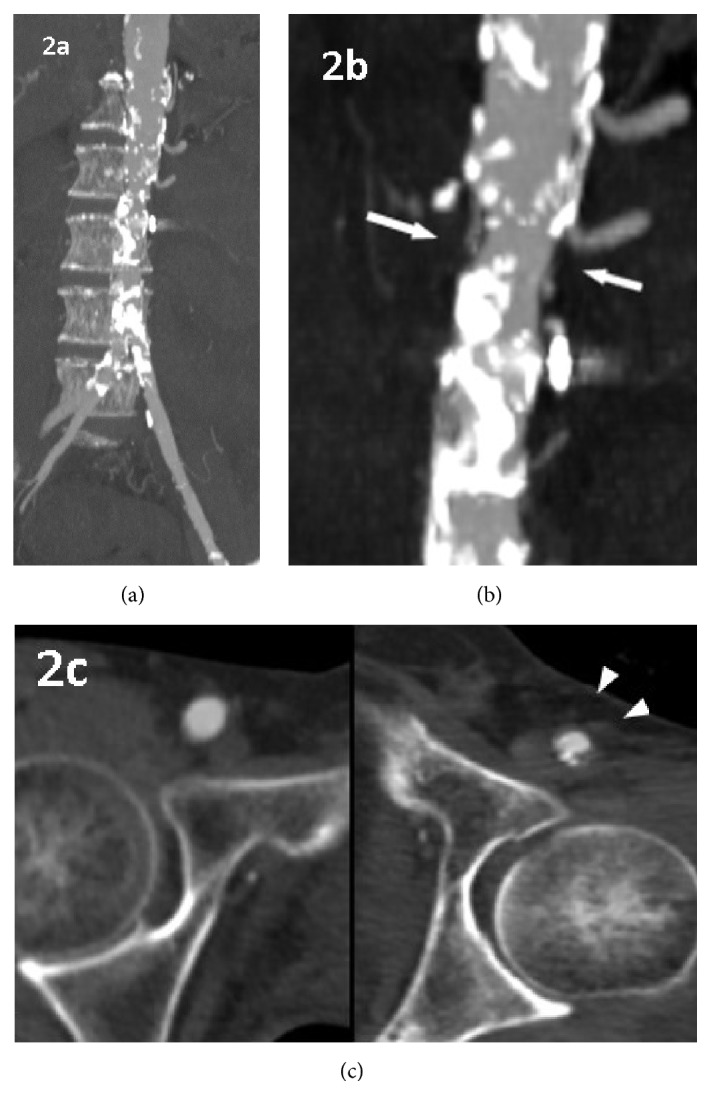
Maximum intensity projection reconstruction of coronal computed tomography angiogram and transverse plane at the level of inguinal region on admission. (a) Diffuse calcification from the abdominal aorta to the left common iliac artery was observed, which was confined to the irradiation field. ((b) and (c)) Irregular stenosis of the abdominal aorta (white arrows) and left femoral artery (arrowhead).
